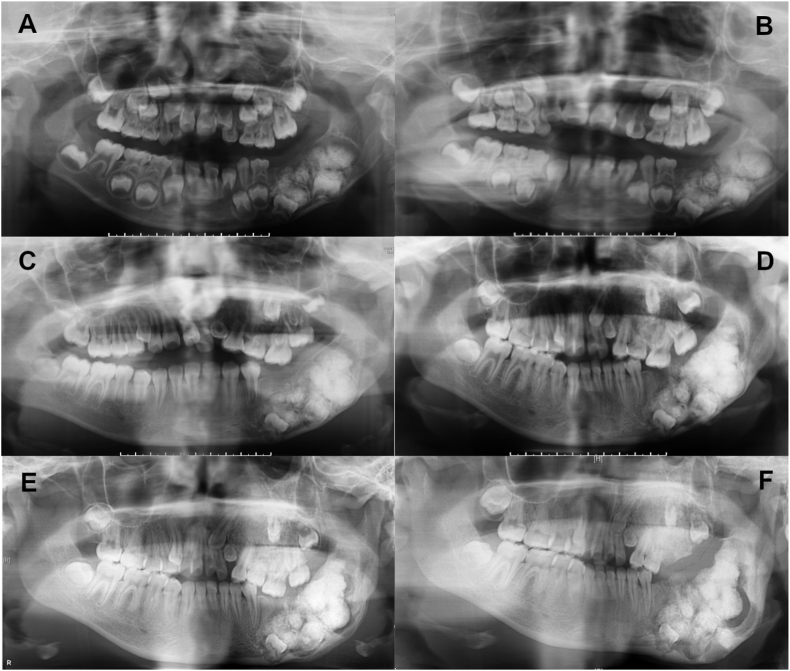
Successive findings in panoramic radiography A (at age 6): A hard tissue lesion in the left mandible was observed, and the left lower second deciduous molar was missing. From B (at age 7), C (at age 11), to D (at age 13): The hard tissues were calcified, and the boundary with the surrounding alveolar bone became indistinct. From E (age 14) to F (age 16): The boundary with the surrounding area gradually became more distinct. The lesion was believed to have originated from the left maxillary bone between B (at age 7) and C (at age 11).
